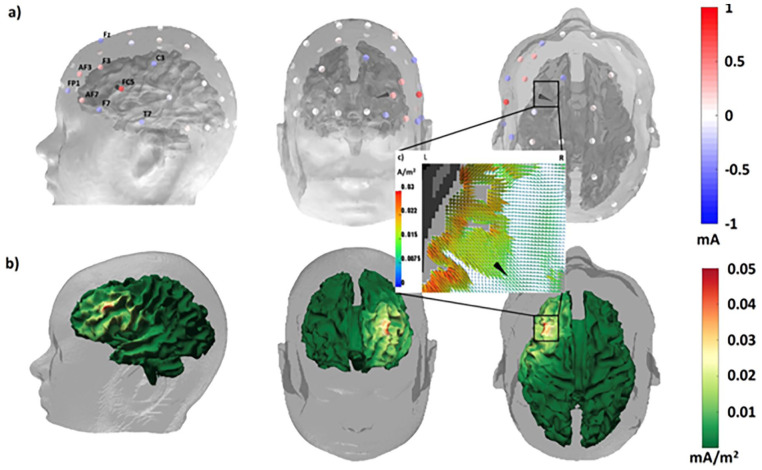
Individually targeted multichannel transcranial direct current stimulation (mc-tDCS) for inhibitory stimulation of a patient with pharmacoresistant epilepsy due to a focal cortical dysplasia located close to the Broca area. (a) Alternating direction method of multipliers (ADMM)-optimized mc-tDCS montage for stimulation of the epileptogenic zone as reconstructed by focal source modeling from electroencephalography (EEG) and magnetoencephalography (MEG) spike activity (black cone). The electrodes are colored by the optimized currents ranging from −1 up to +1 mA. The total sum of the injected currents is equal to 2 mA fulfilling safety constraints. The optimized mc-tDCS current density distribution visualized (b) on the cortical surface and (c) on a zoomed axial magnetic resonance imaging (MRI) view in the target area. The colored brain surface of the patient represents the distribution of the current density measured in mA/m^2^.
